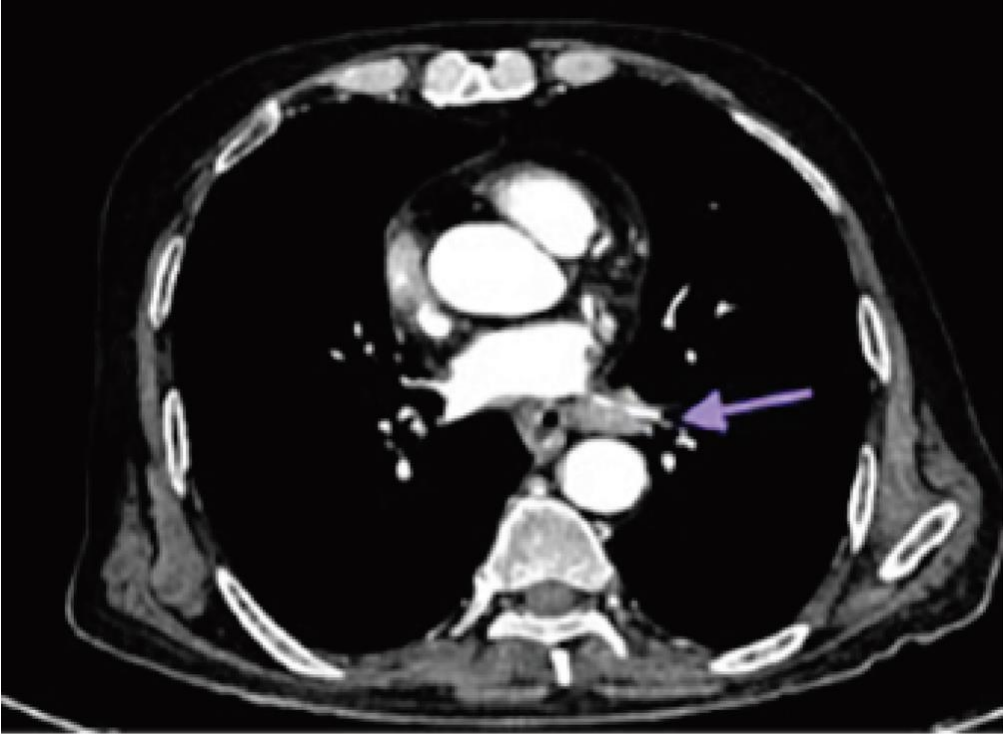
Contrast enhanced computed tomography of thorax showing multiple conglomerated, heterogeneously enhancing mediastinal lymph nodes (arrow).
